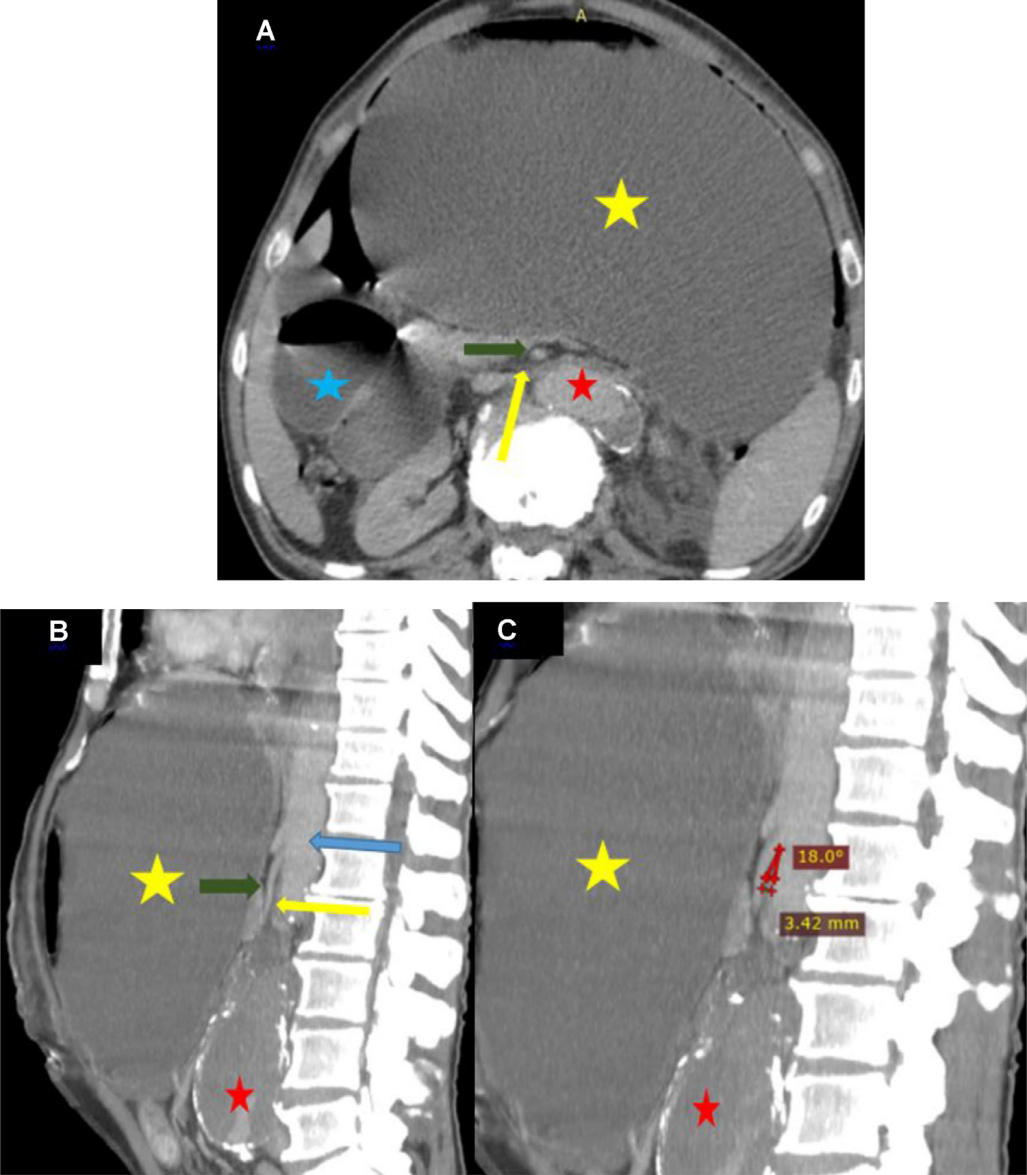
Abdominal CT Angiography: Axial view (a) and coronal reconstructions (b and c). The angle between the superior mesenteric artery (SMA) (green arrow) and the abdominal aorta (blue arrow) is markedly reduced to 18°, with a decreased aorto-mesenteric distance (3.42 mm). A fusiform suprarenal abdominal aortic aneurysm (red star) is present and may have contributed to the reduction of the aorto-mesenteric angle. This anatomical configuration leads to severe gastric and duodenal distension (yellow and blue stars), responsible for anterior extrinsic compression of the third portion of the duodenum (D3) (yellow arrow), which is trapped between the SMA and the aorta. The resulting duodenal obstruction is therefore secondary to distension-related mechanical compression rather than a direct mass effect from the aneurysm.
